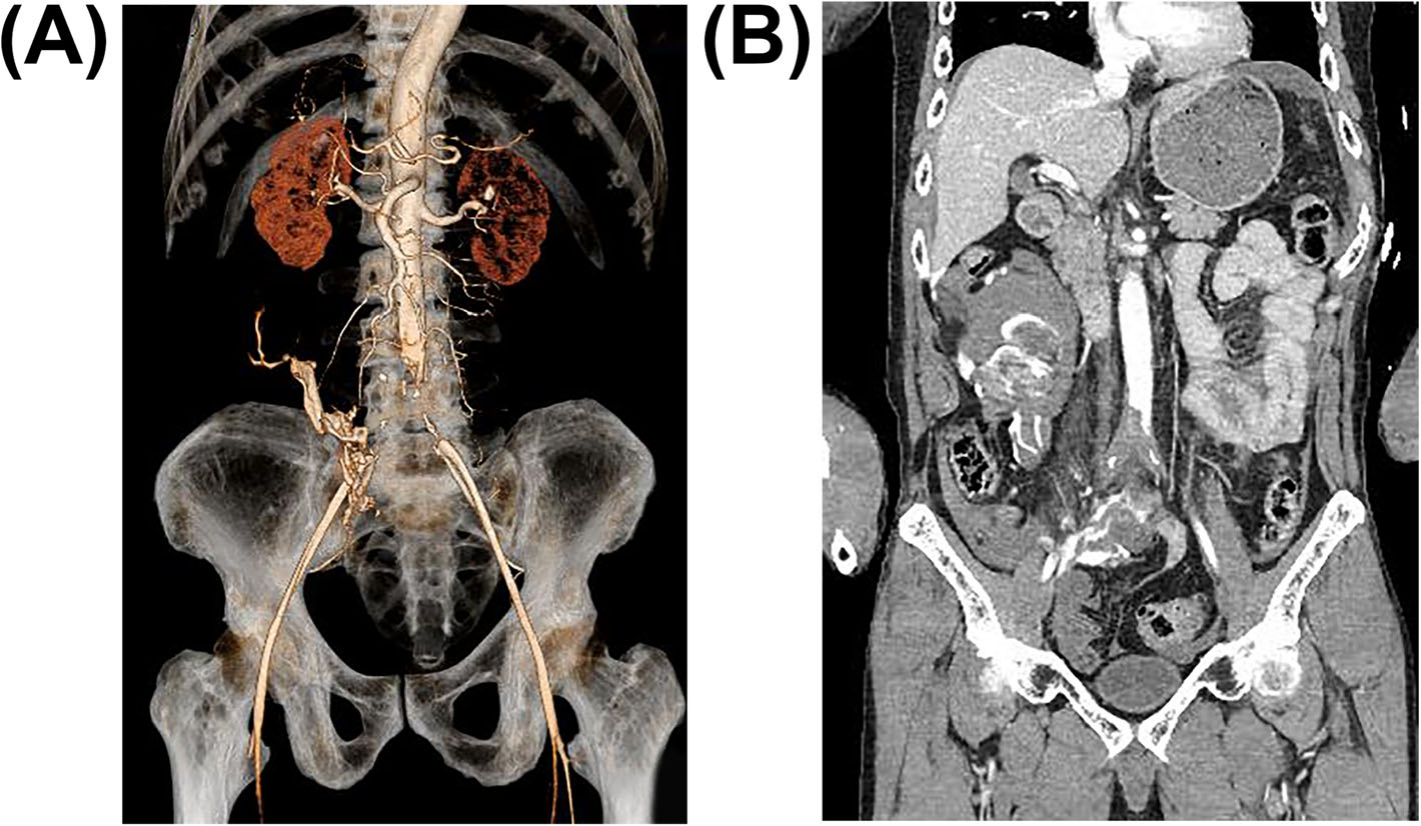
Coronal computed tomographic (CT) reconstructions in arterial phase images. A Complete occlusion just above the bifurcation of the abdominal aorta on 3D-CT image. In particular, the collateral blood pathway to the right external iliac artery can be seen. B Coronal contrast-enhanced CT images show disruption of blood flow due to bilateral thrombi at the aortic bifurcation and distal blood flow
